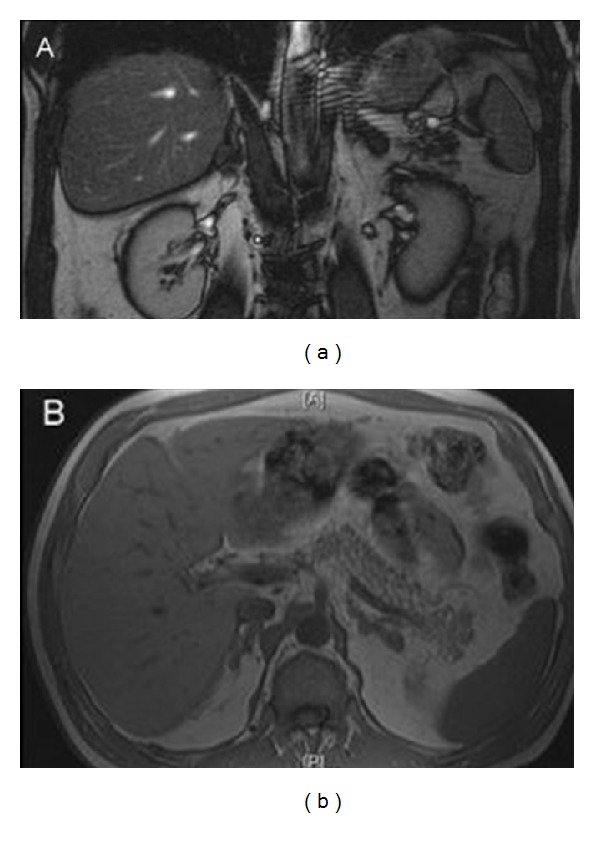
Magnetic resonance imaging of the upper abdomen showing asymmetric, bilateral enlargement of the adrenal glands.
